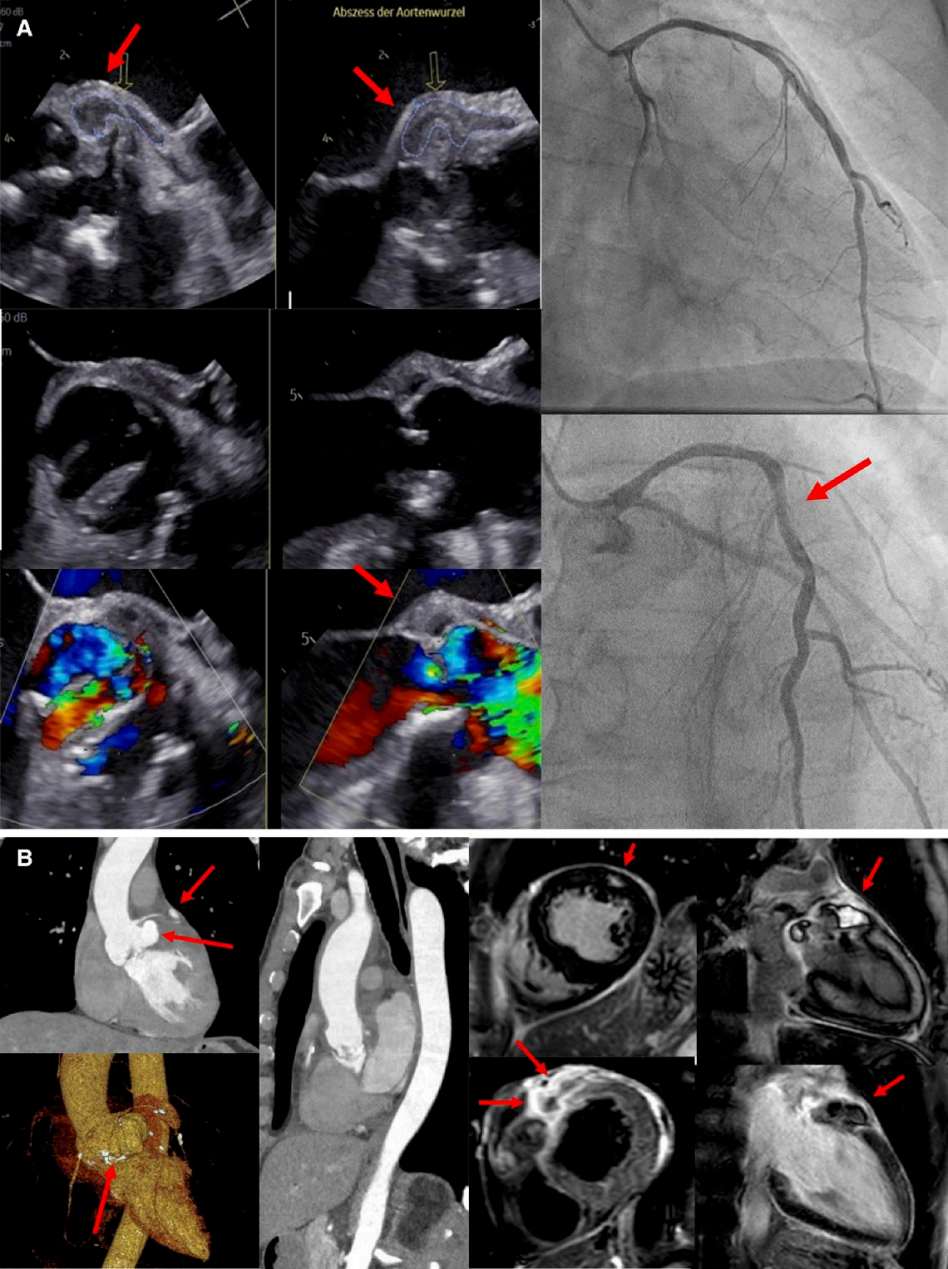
(A)* Left side: Transoesophageal echocardiography demonstrating the aortic root abscess. On the right side: Coronary angiography showing normal left anterior descending (upper picture) and 60% stenosis of the circumflex artery (lower picture), most likely causing the, fake pectanginous symptoms due to the abscess additionally pressing on the artery. *(B)* Multimodal imaging methods presenting the massive aortic root abscess expanding from the anterior cusp of the bicuspid valve to the anterior basal ventricular myocardium. Left side: Computed tomography angiography demonstrating the large aortic root abscess with an axial size of ∼35 × 30 × 26 mm with expansion from the anterior cusp of the bicuspid aortic valve to the anterior basal ventricular myocardium. Right side, *top* row: Late gadolinium enhancement short-axis view showing another abscess formation at the end of the intramyocardial road of pus inside the lateral midventricular wall, firstly interpreted as microembolic lesion (short-axis view, second picture from the upper right). On the right side: a magnitude image for better visualization of the aortic root abscess (2-CH view). *Bottom* row: a T_2_ SA demonstrating myocardial oedema. Left side: Late gadolinium enhancement 2-CH view with the intramyocardial abscess.
